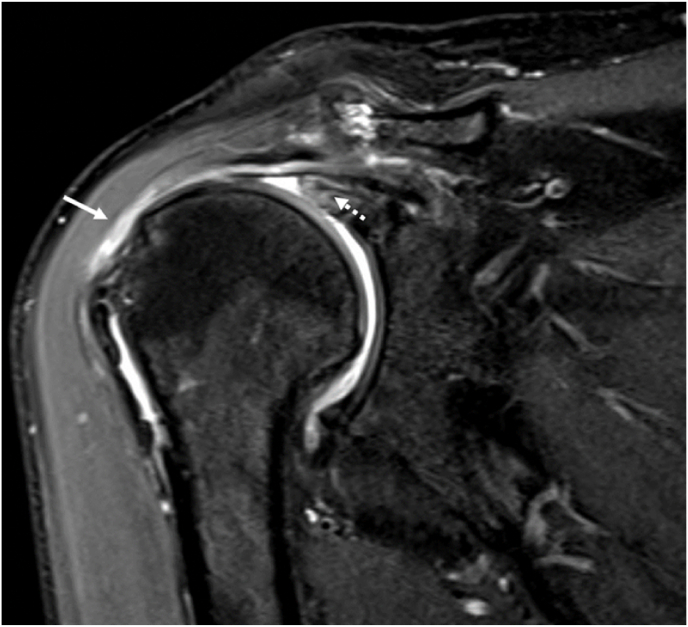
Coronal proton density fat-saturation MR image demonstrates partial under surface tear and fibers fraying at the myotendinous junction of the middle portion of the deltoid muscle (white arrow), which is in close proximity to the humeral greater tuberosity, in another patient presented with a chronic moderate retracted full-thickness tear of the supraspinatus tendon (dotted white arrow).
